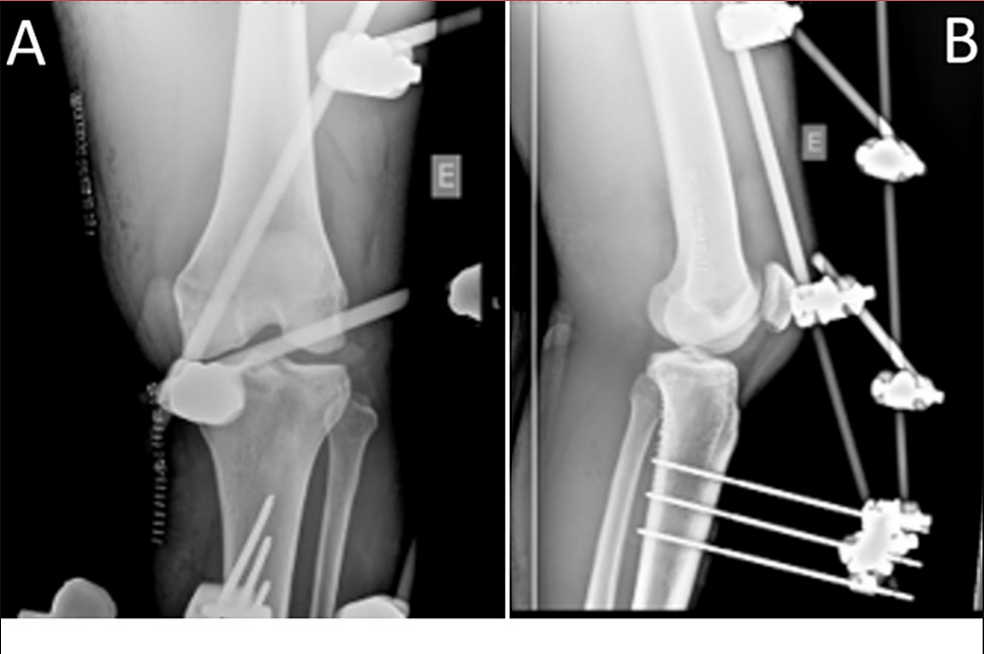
Radiograph of knee joint after external fixator application. A – posteroanterior view; B- lateral view
